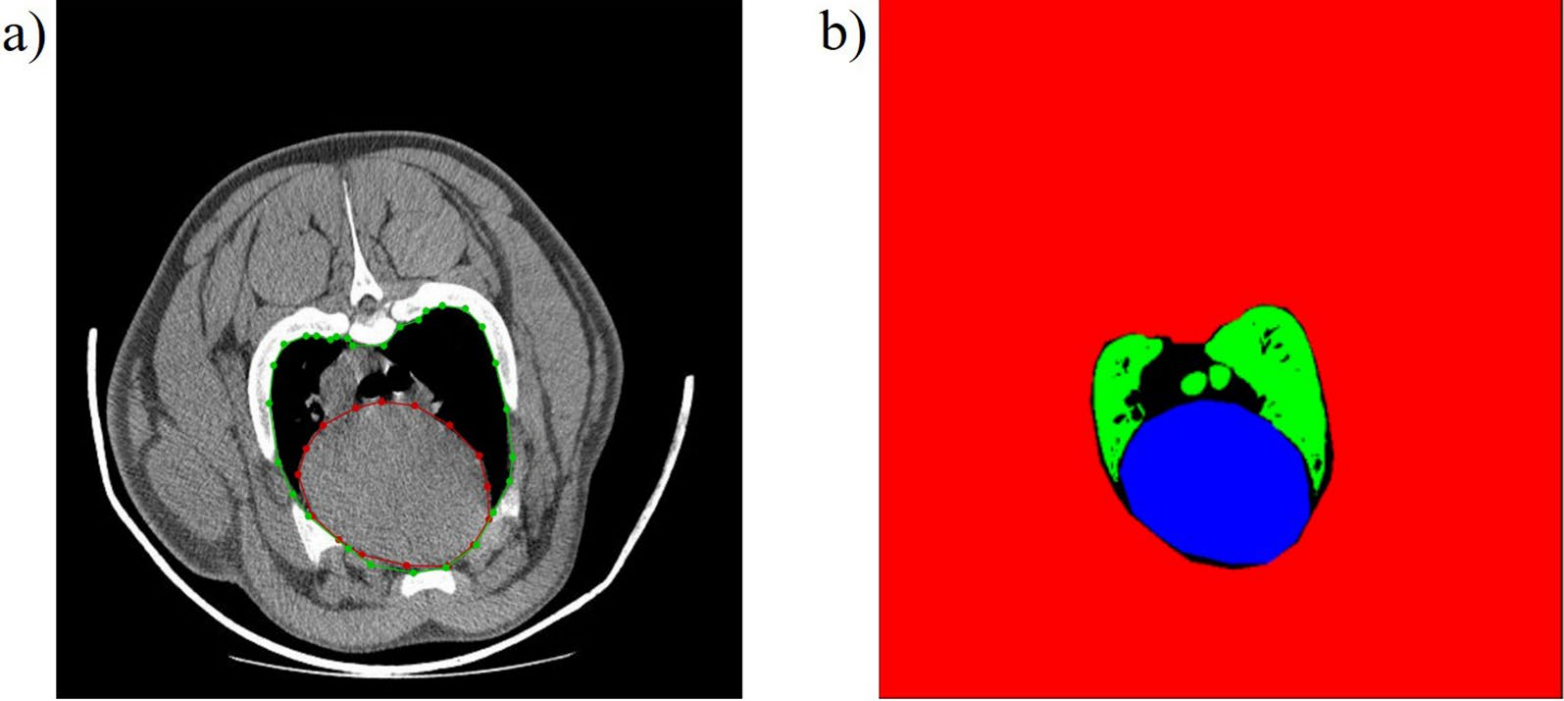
Manual annotation of CT-image. (a) Polygons covering the heart only (red) and heart and lung area combined (green). (b) Final labelling of heart (in blue), the lungs (in green) and background (in red). The region coloured in black is not assigned to any class.
